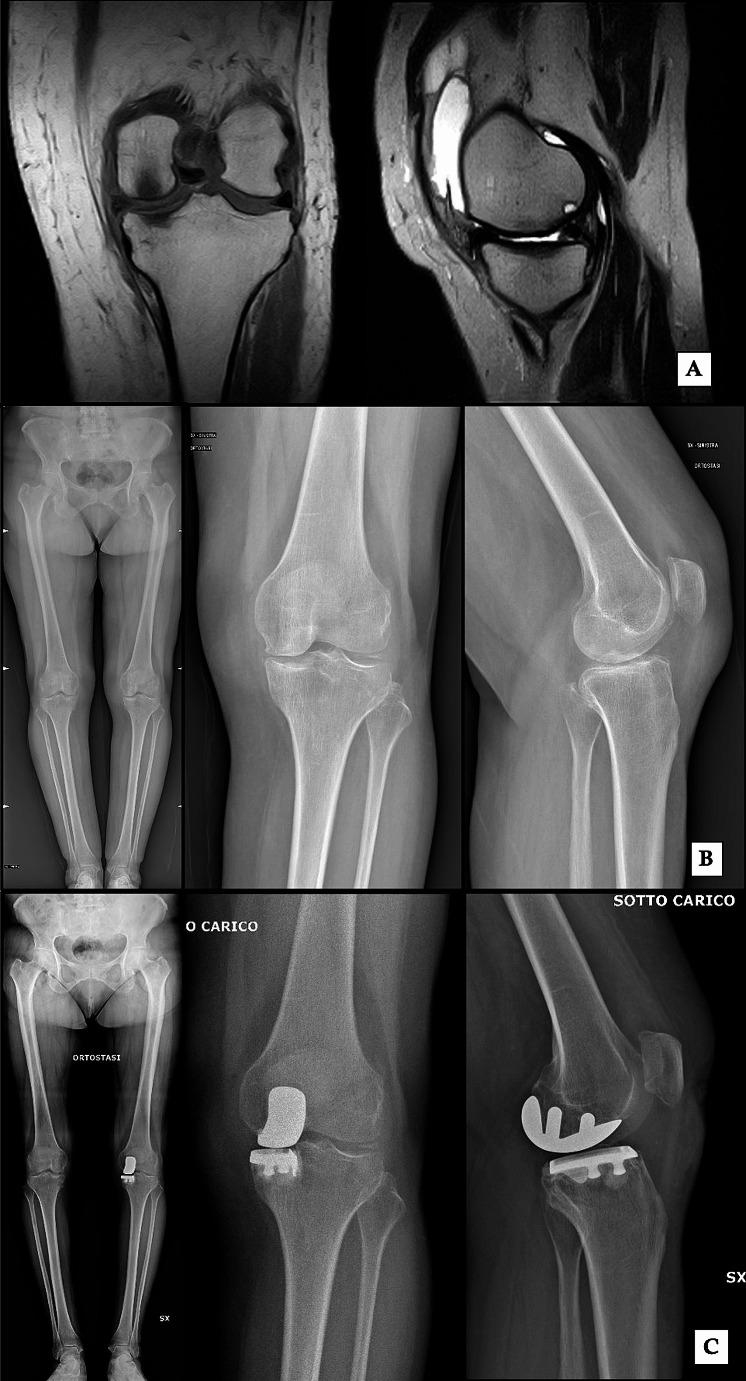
BME of the medial femoral condyle and subchondral cyst in a 70 year female patient at T2 MRI sequences (A) and X-Ray before (B) and after (C) medial unicompartmental knee arthroplasty
